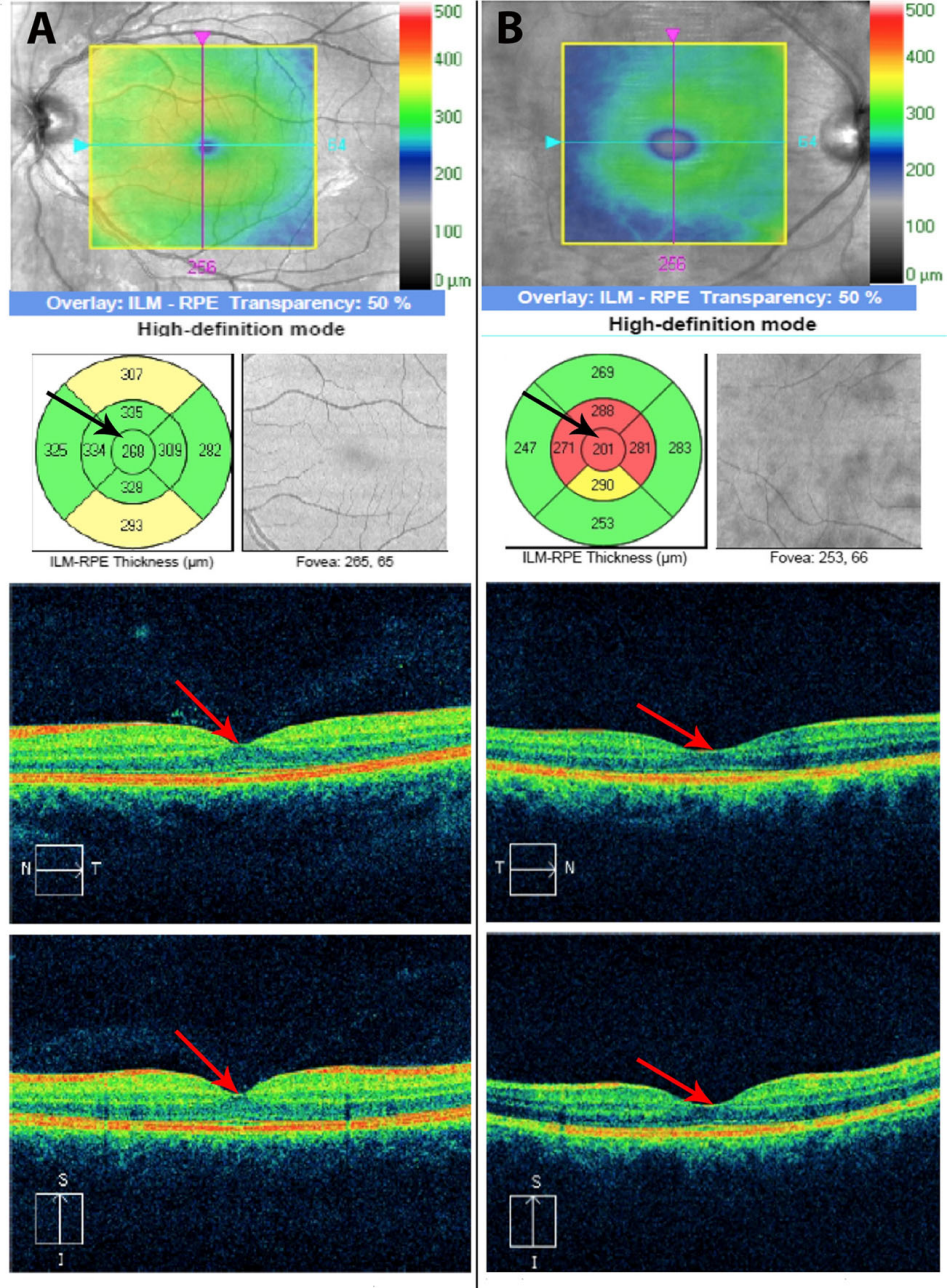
a Physiological central macular thickness 268 μm for MRI negative WD patient (arrows show central subfield thickness); b Pathological central macular thickness 201 μm for MRI positive WD patient (arrows show central subfield thickness)
